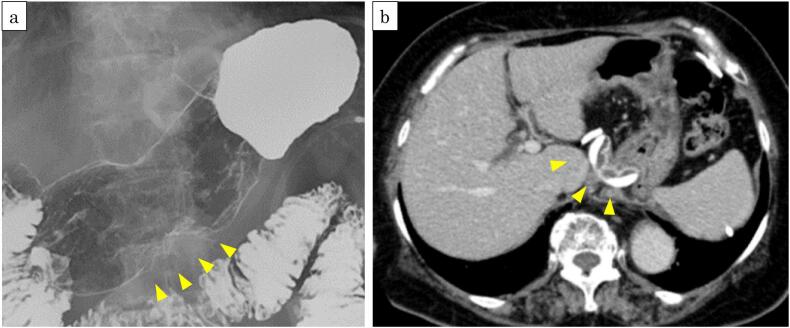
a: Upper gastrointestinal contrast imaging revealed a trapezoidal deformity mainly on the posterior wall., b: Computed tomography showed adjustable banding was observed in the upper gastric body. There was no obvious distant metastasis or lymph node metastasis.
